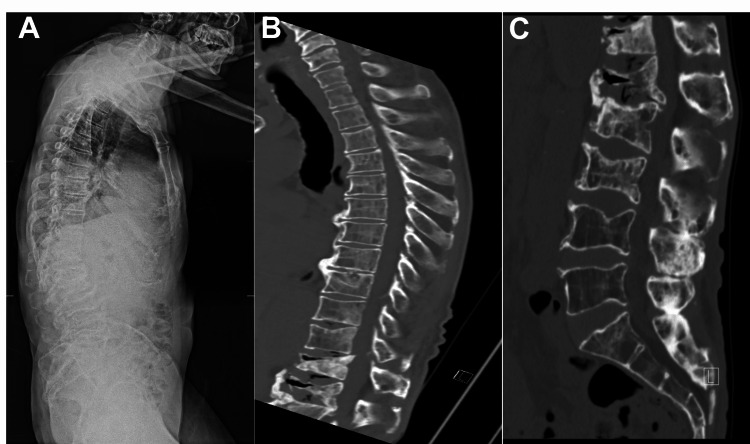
Scoliosis standing X-ray shows significant positive balance with pronounced thoracic kyphosis (A). Sagittal CT without contrast show T12, L1, L2, and L3 compression fractures with severe height loss seen at the L1 body (B & C).
